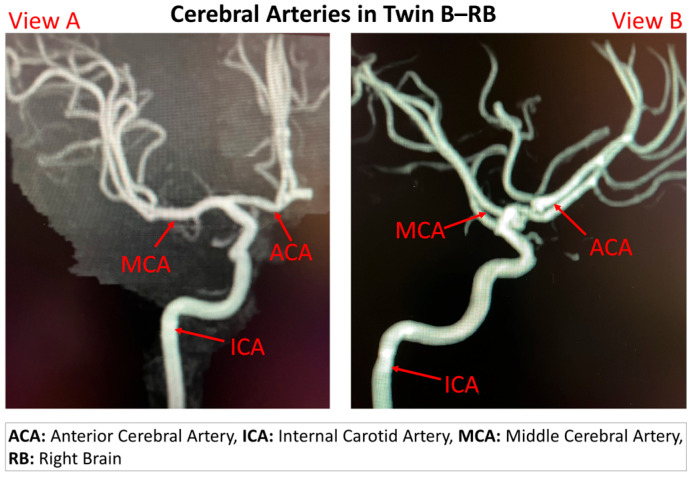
Cerebral artery system in twin B-RB based on collected MRI images from two viewing perspectives (i.e., view A and view B).
